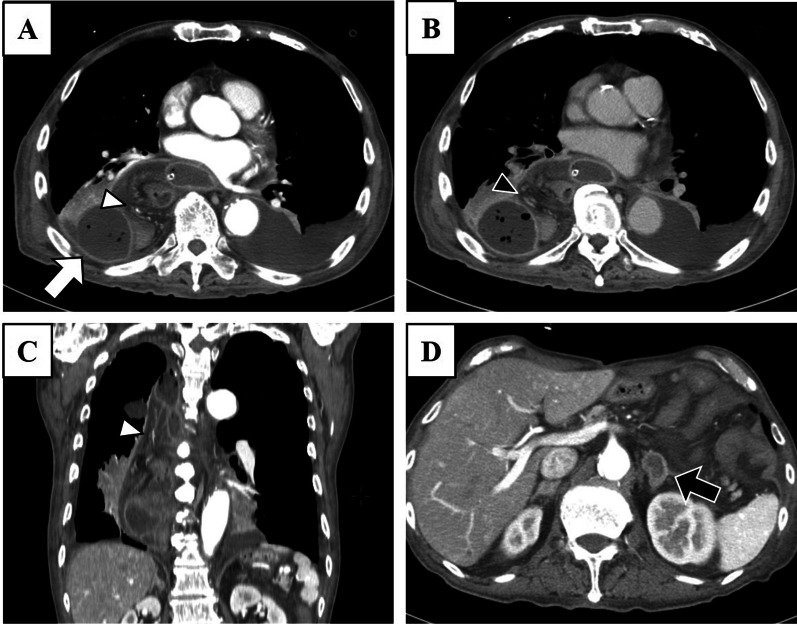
Contrast-enhanced computed tomography (CT) on the 9th hospital day. A Contrast-enhanced CT confirmed the arterial flow in the mesentery of the reconstructed jejunum (white arrowhead) and the intrathoracic abscess formation was organized (white arrow). B In the venous phase, sufficient venous flow was confirmed without disruption or thrombus in the mesenteric vessels of the reconstructed jejunum that was elevated in the thoracic cavity (black arrowhead). The arterial and venous flows in mesentery of the reconstructed jejunum were maintained. C Sagittal CT image showed sufficient arterial blood flow in the reconstructed jejunum that was elevated in the thoracic cavity (white arrowhead). D Metastatic left adrenal tumor derived from the remnant gastric cancer could be seen on the CT image
